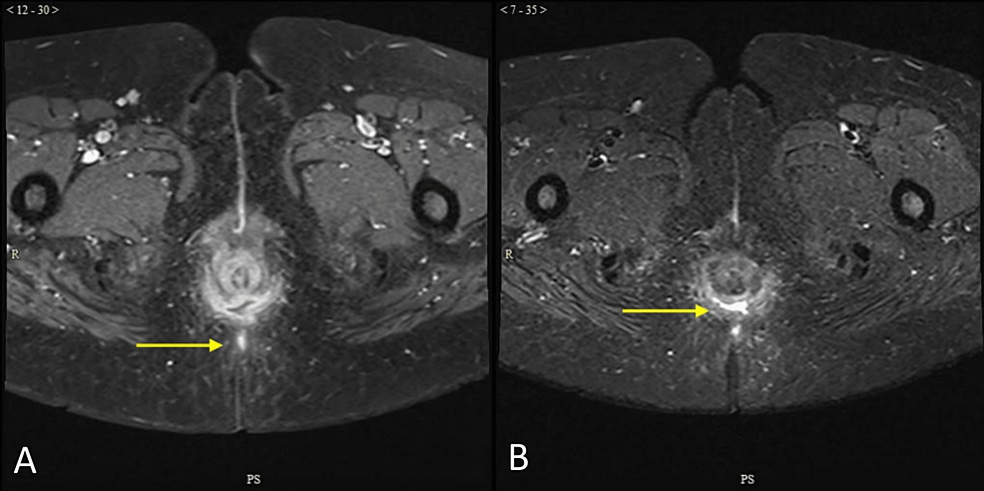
Horseshoe abcess on a T1C with the arrow indicating the tract (A) and STIR with the arrow indicating the abscess (B) T1C: post-contrast T1-weighted imaging; STIR: Short Tau Inversion Recovery
